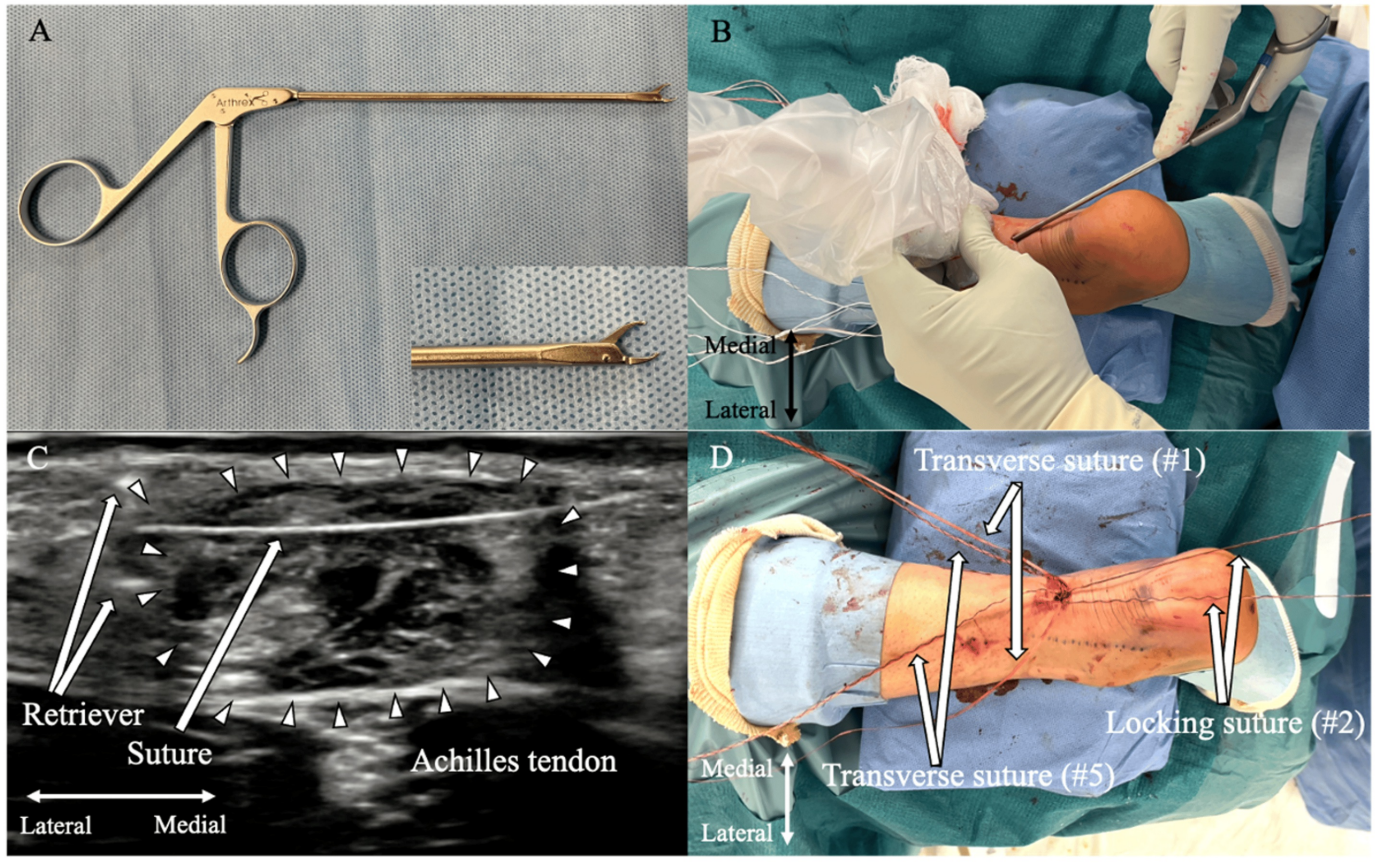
Suture retrieval from the proximal Achilles tendon stump under ultrasound guidance, followed by locking suture placement A: Suture retriever tool; B: External view during ultrasound-guided suture retrieval; C: Ultrasound visualization of suture retrieval; D: Final view after retrieval and locking suture placement Image source: Authors
